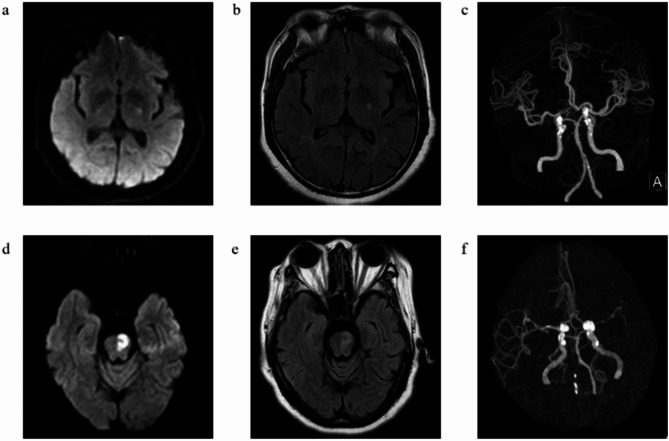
Site of CI and intracranial vascular images in 2 patients a-b. DWI imaging and FLAIR imaging showed that the left thalamic punctate high signal of case 1 was suggestive of a new infarction; c. Intracranial CTA images at the same level of case 1 demonstrated his calcified plaques in the siphon segments of the internal carotid arteries bilaterally; d-e. DWI imaging and FLAIR imaging showed that the left ventral posterolateral pons revealed patchy high signal of case 2, which indicated the site of new infarction; f. Intracranial CTA images of case 2 hinted multiple intracranial stenosis of the patient, with severe stenosis of the right vertebral artery
